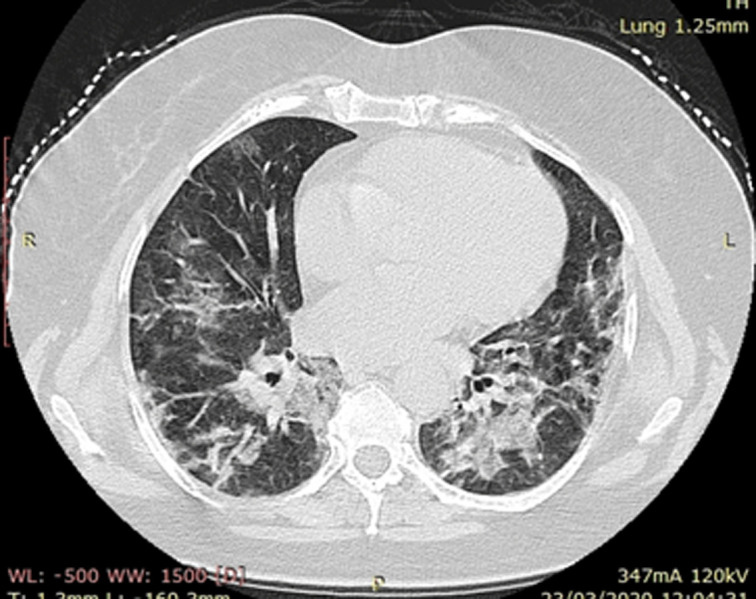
admission chest computed tomography
